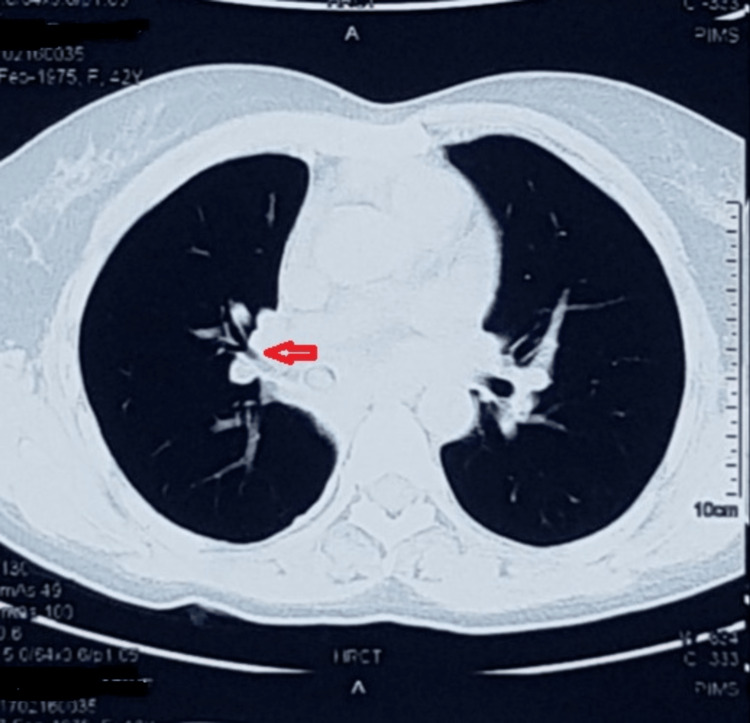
HRCT of the chest showing an endoluminal mass in the right bronchus intermedius. HRCT, high-resolution computed tomography
